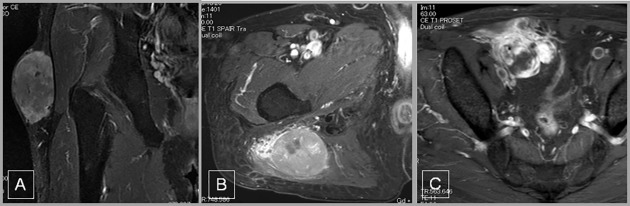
Infiltrative growth patterns on MRI. (a) pushing-type (b) focal-type and (c) diffuse-type.
